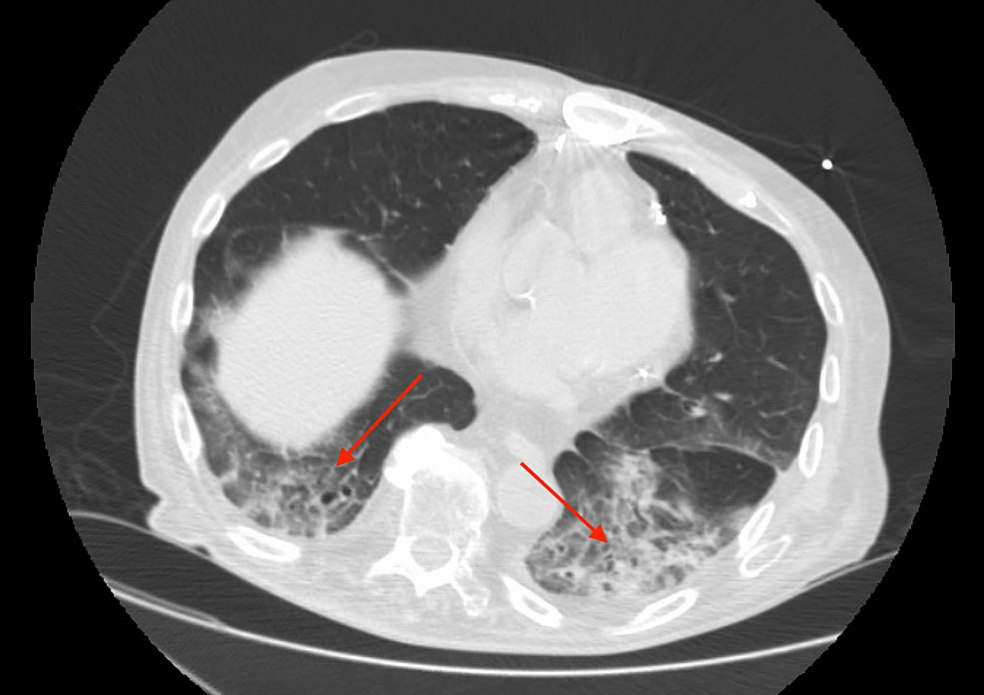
Chest CT showing worsening of infiltrates, raising suspicion of organizing pneumonia (arrows)
